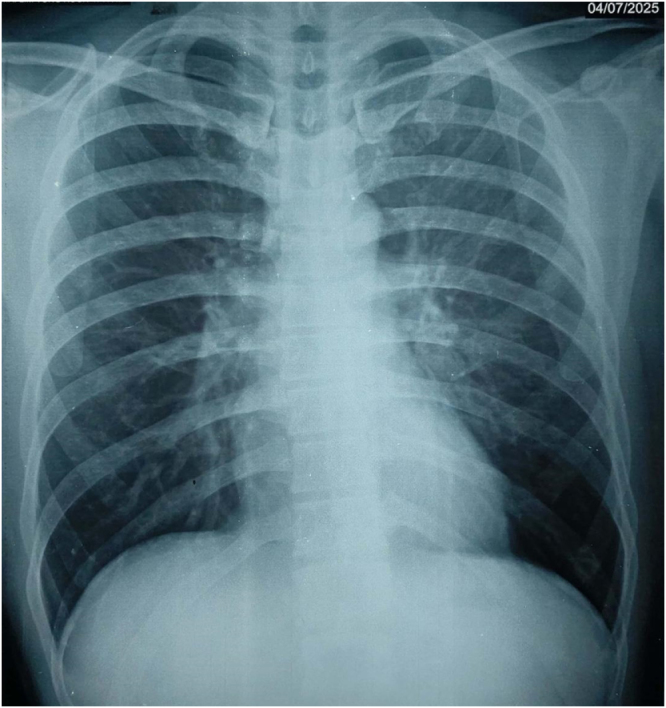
Normal chest radiograph of the patient performed at the time of presentation.
